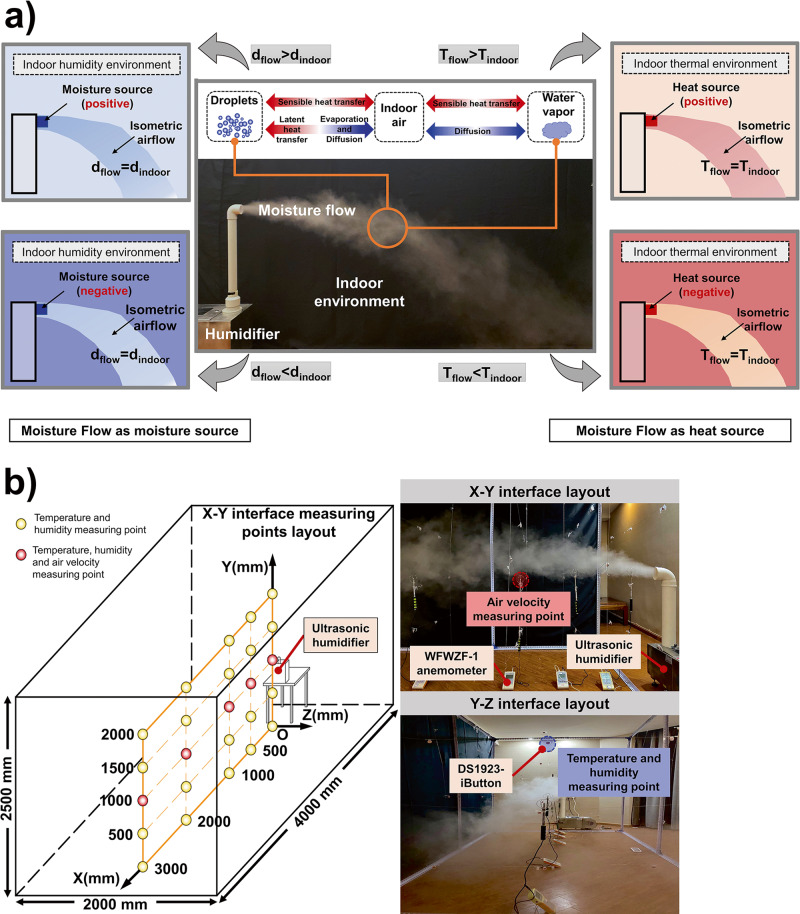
Feasibility Verification Experiment. Feasibility verification experiment of Rate of Humidity Contribution Change ($${{{{{{\rm{CRI}}}}}}}_{({{{{{\rm{H}}}}}})}^{{{{{{\rm{t}}}}}}}$$) and Rate of Indoor Climate Contribution Change ($${{{{{{\rm{CRI}}}}}}}_{({{{{{\rm{C}}}}}})}^{{{{{{\rm{t}}}}}}}$$) by an ultrasonic humidifier under various source parameters and environmental conditions. a Moisture flow as a moisture and heat source. When a humidifier is used to deliver moisture to a room, the airflow (air humidity d_flow_, air temperature T_flow_) generated by the device exchanges the moisture and heat with the indoor air (air humidity d_indoor_, air temperature T_indoor_) simultaneously, which can be regarded as a moisture source for the humidity field and a heat source for the temperature field. b Layout of the measuring points and measurement facilities. The air temperature and humidity measuring points are arranged in the XOY plane (X = 0, 500, 1000, 2000, 3000 mm, Y = 0, 500, 1000, 1500 and 2000 mm), and the air velocity measuring points are arranged at X = 0, 500, 1000, 2000 and 3000 mm along the outlet axis of the source. The sampling interval is set to 1 min.
